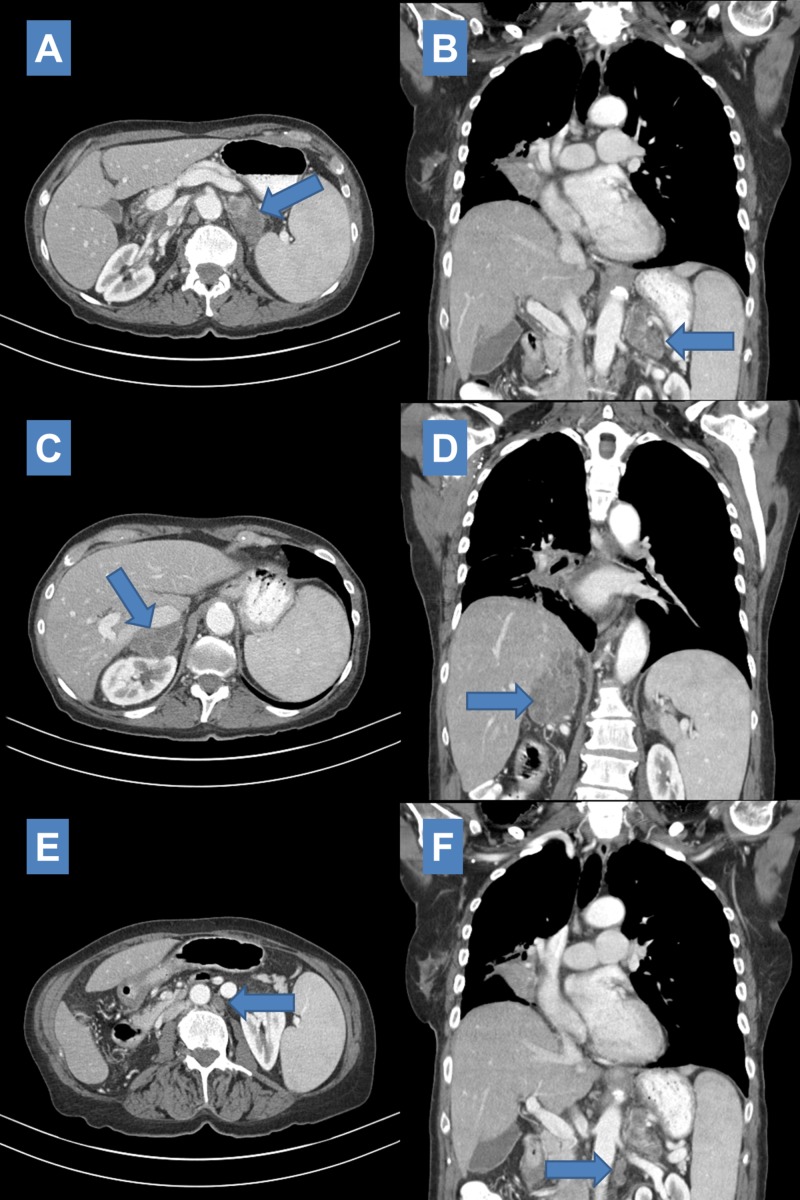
Three-month post salvage SBRT CT imaging scan showing stable size of left (4.4 cm x 2.6 cm SBRT: Stereotactic body radiation therapy; CT: Computed tomography.
